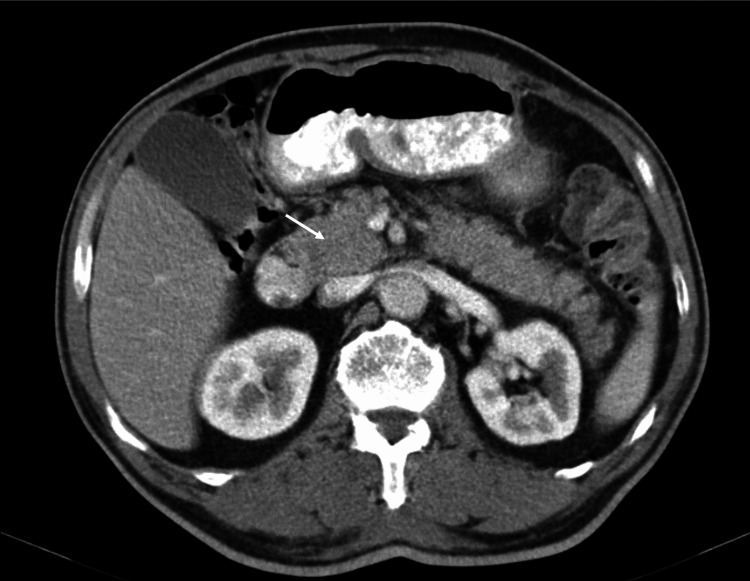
Abdominal CT scan The white arrow points towards a slightly spherical appearance of the cephalic pancreatic region.
